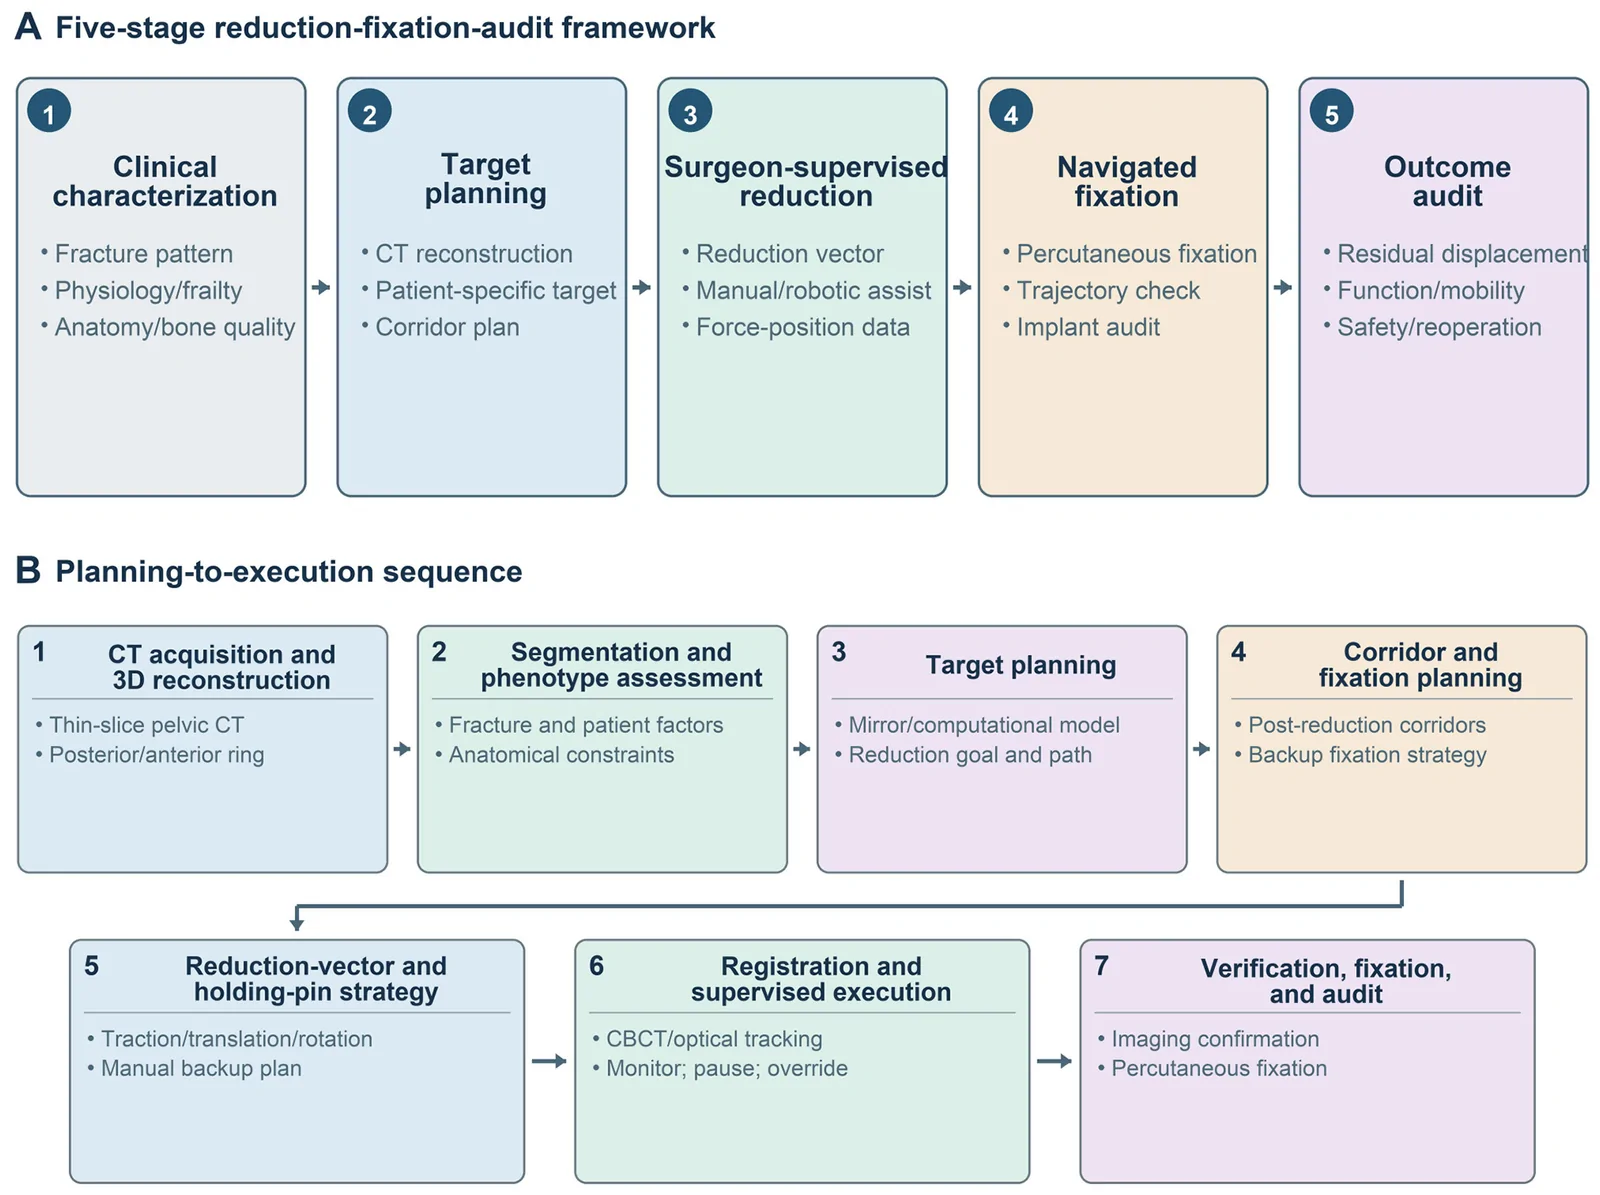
Patient-specific reduction-fixation-audit framework and surgeon-supervised planning-to-execution sequence for pelvic ring surgery. (A) links clinical characterization, target planning, surgeon-supervised reduction, navigated fixation, and outcome audit. (B) expands the planning-to-execution sequence from CT acquisition and phenotype assessment to target/corridor planning, reduction-vector and holding-pin strategy, registration, monitored execution with pause or override, imaging verification, fixation, and postoperative audit. The framework is conceptual and is not a device-specific protocol or clinical guideline.
